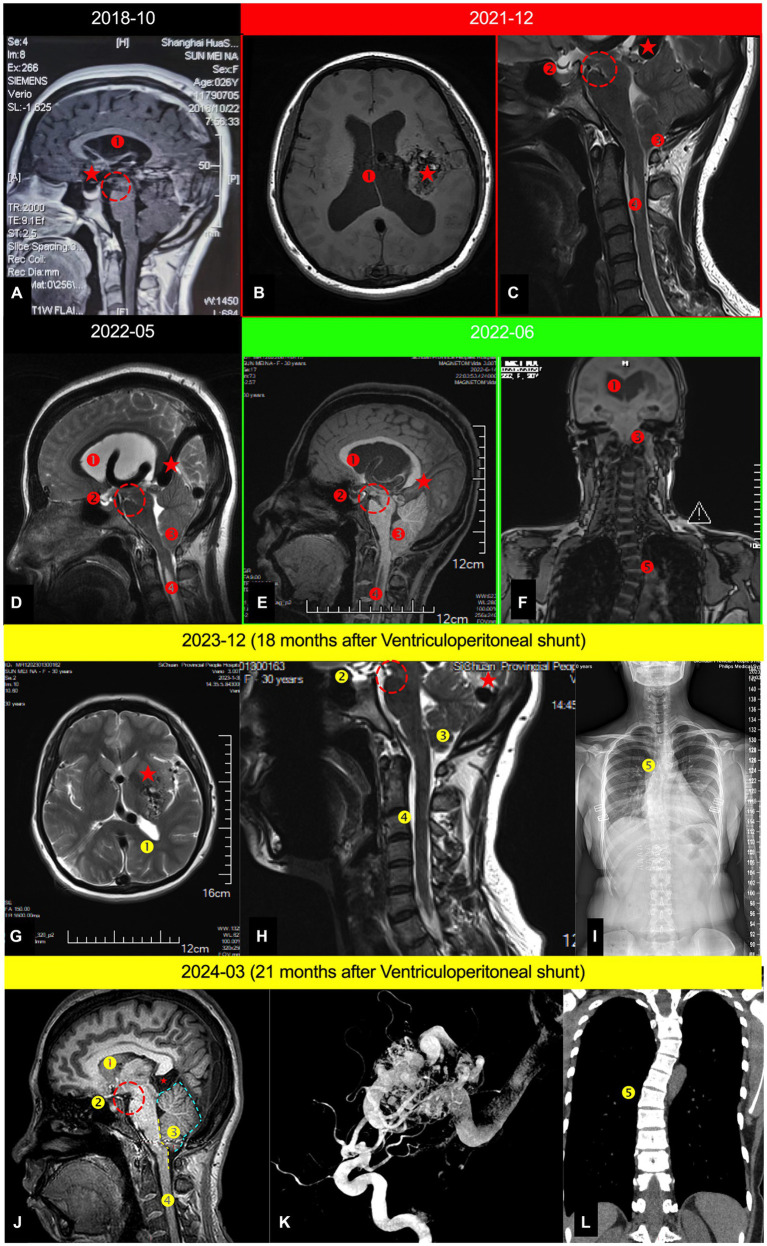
Serial cranial MRI examinations depicting the entire treatment and postoperative follow-up process of the patient; (A) Mid-sagittal cranial MRI in December 2018; (B) Axial cranial MRI in December 2021; (C). Mid-sagittal cervical MRI in December 2021; (D) Mid-sagittal cranio-cervical MRI in May 2022; (E) Mid-sagittal cranio-cervical MRI in June 2022; (F) Coronal spinal MRI in June 2022; (G) Axial cranial MRI in December 2023 (18 months postoperative); (H) Mid-sagittal cervical MRI (18 months postoperative); (I) Full spine X-ray (18 months postoperative). (J) Mid-sagittal cervical MRI (21 months postoperative); (K) The digital subtraction angiography(21 months postoperative); (L) Coronal spinal MRI in March 2024; ⁎: AVM; ①. Hydrocephalus; ②. Empty sella syndrome; ③. Secondary CMI (Chiari malformation type 1); ④. Spinal syrinx; ⑤. Scoliosis; Yellow dashed arrow. The CSF shunt tube. Red dashed circle. Indicates the sign of midbrain sagging, referred to as the mamillopontine distance, with the specific measurement method available ④; Blue dashed line in Figure 1J represents the swollen boundary of the cerebellum; The yellow dashed line in Figure 1J delineates the boundary where the brainstem is tortuous and compressed.
